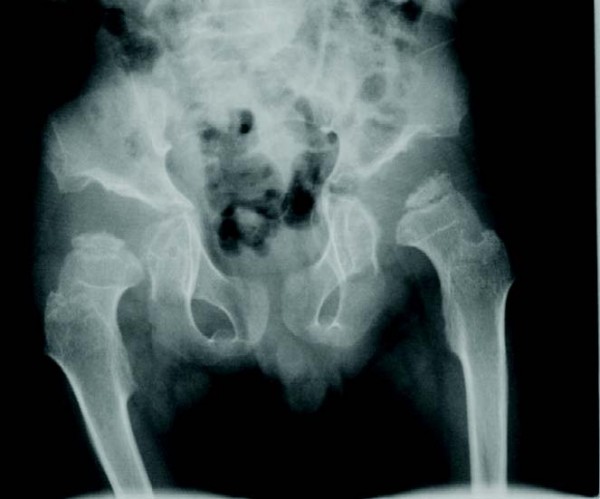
Rapidly progressing 9-year old female MPS VI patient: radiograph of hip showing dysplastic femoral head and severe hip dysplasia.
